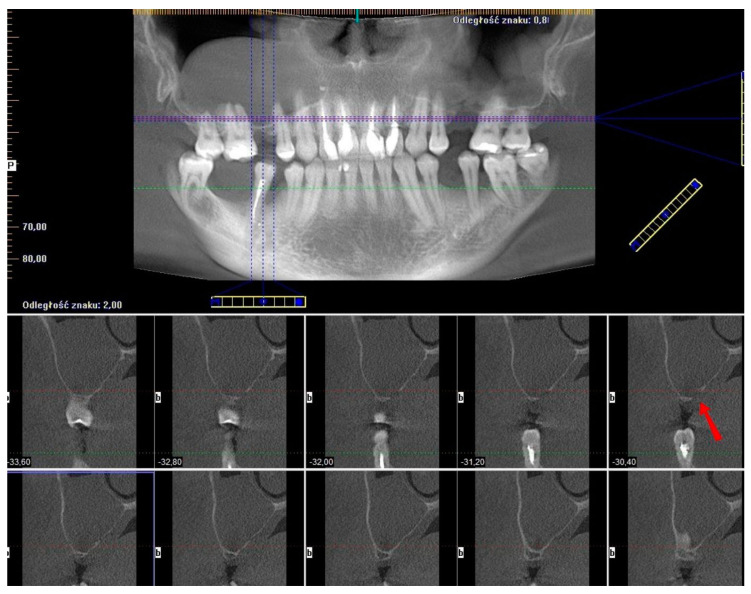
Croco Eye Technique—final version. Screenshot of the pre-op CBCT scans with a visible radiopaque lesion in the right maxillary sinus, insufficient alveolar ridge height, and noncontinuous bone of the sinus floor due to past oroantral communication (OAC) (red arrow).
